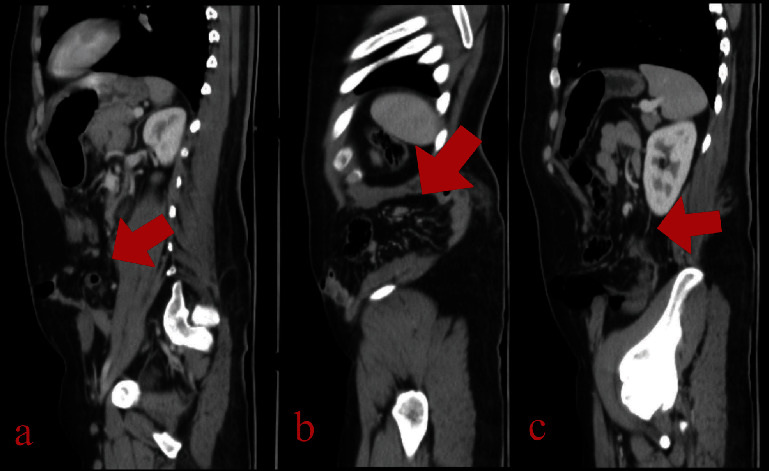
CT abdomen-pelvis imaging: (a–c) lateral slices, demonstrating the TAWH.
